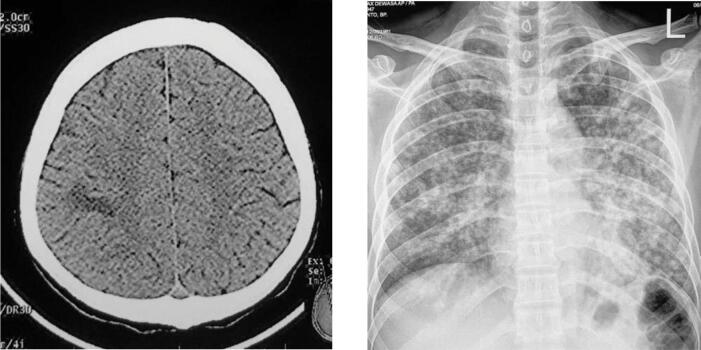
The CT Scan with hypodense lesion and the chest X-ray showed multiple uniform nodule in both lungs.
